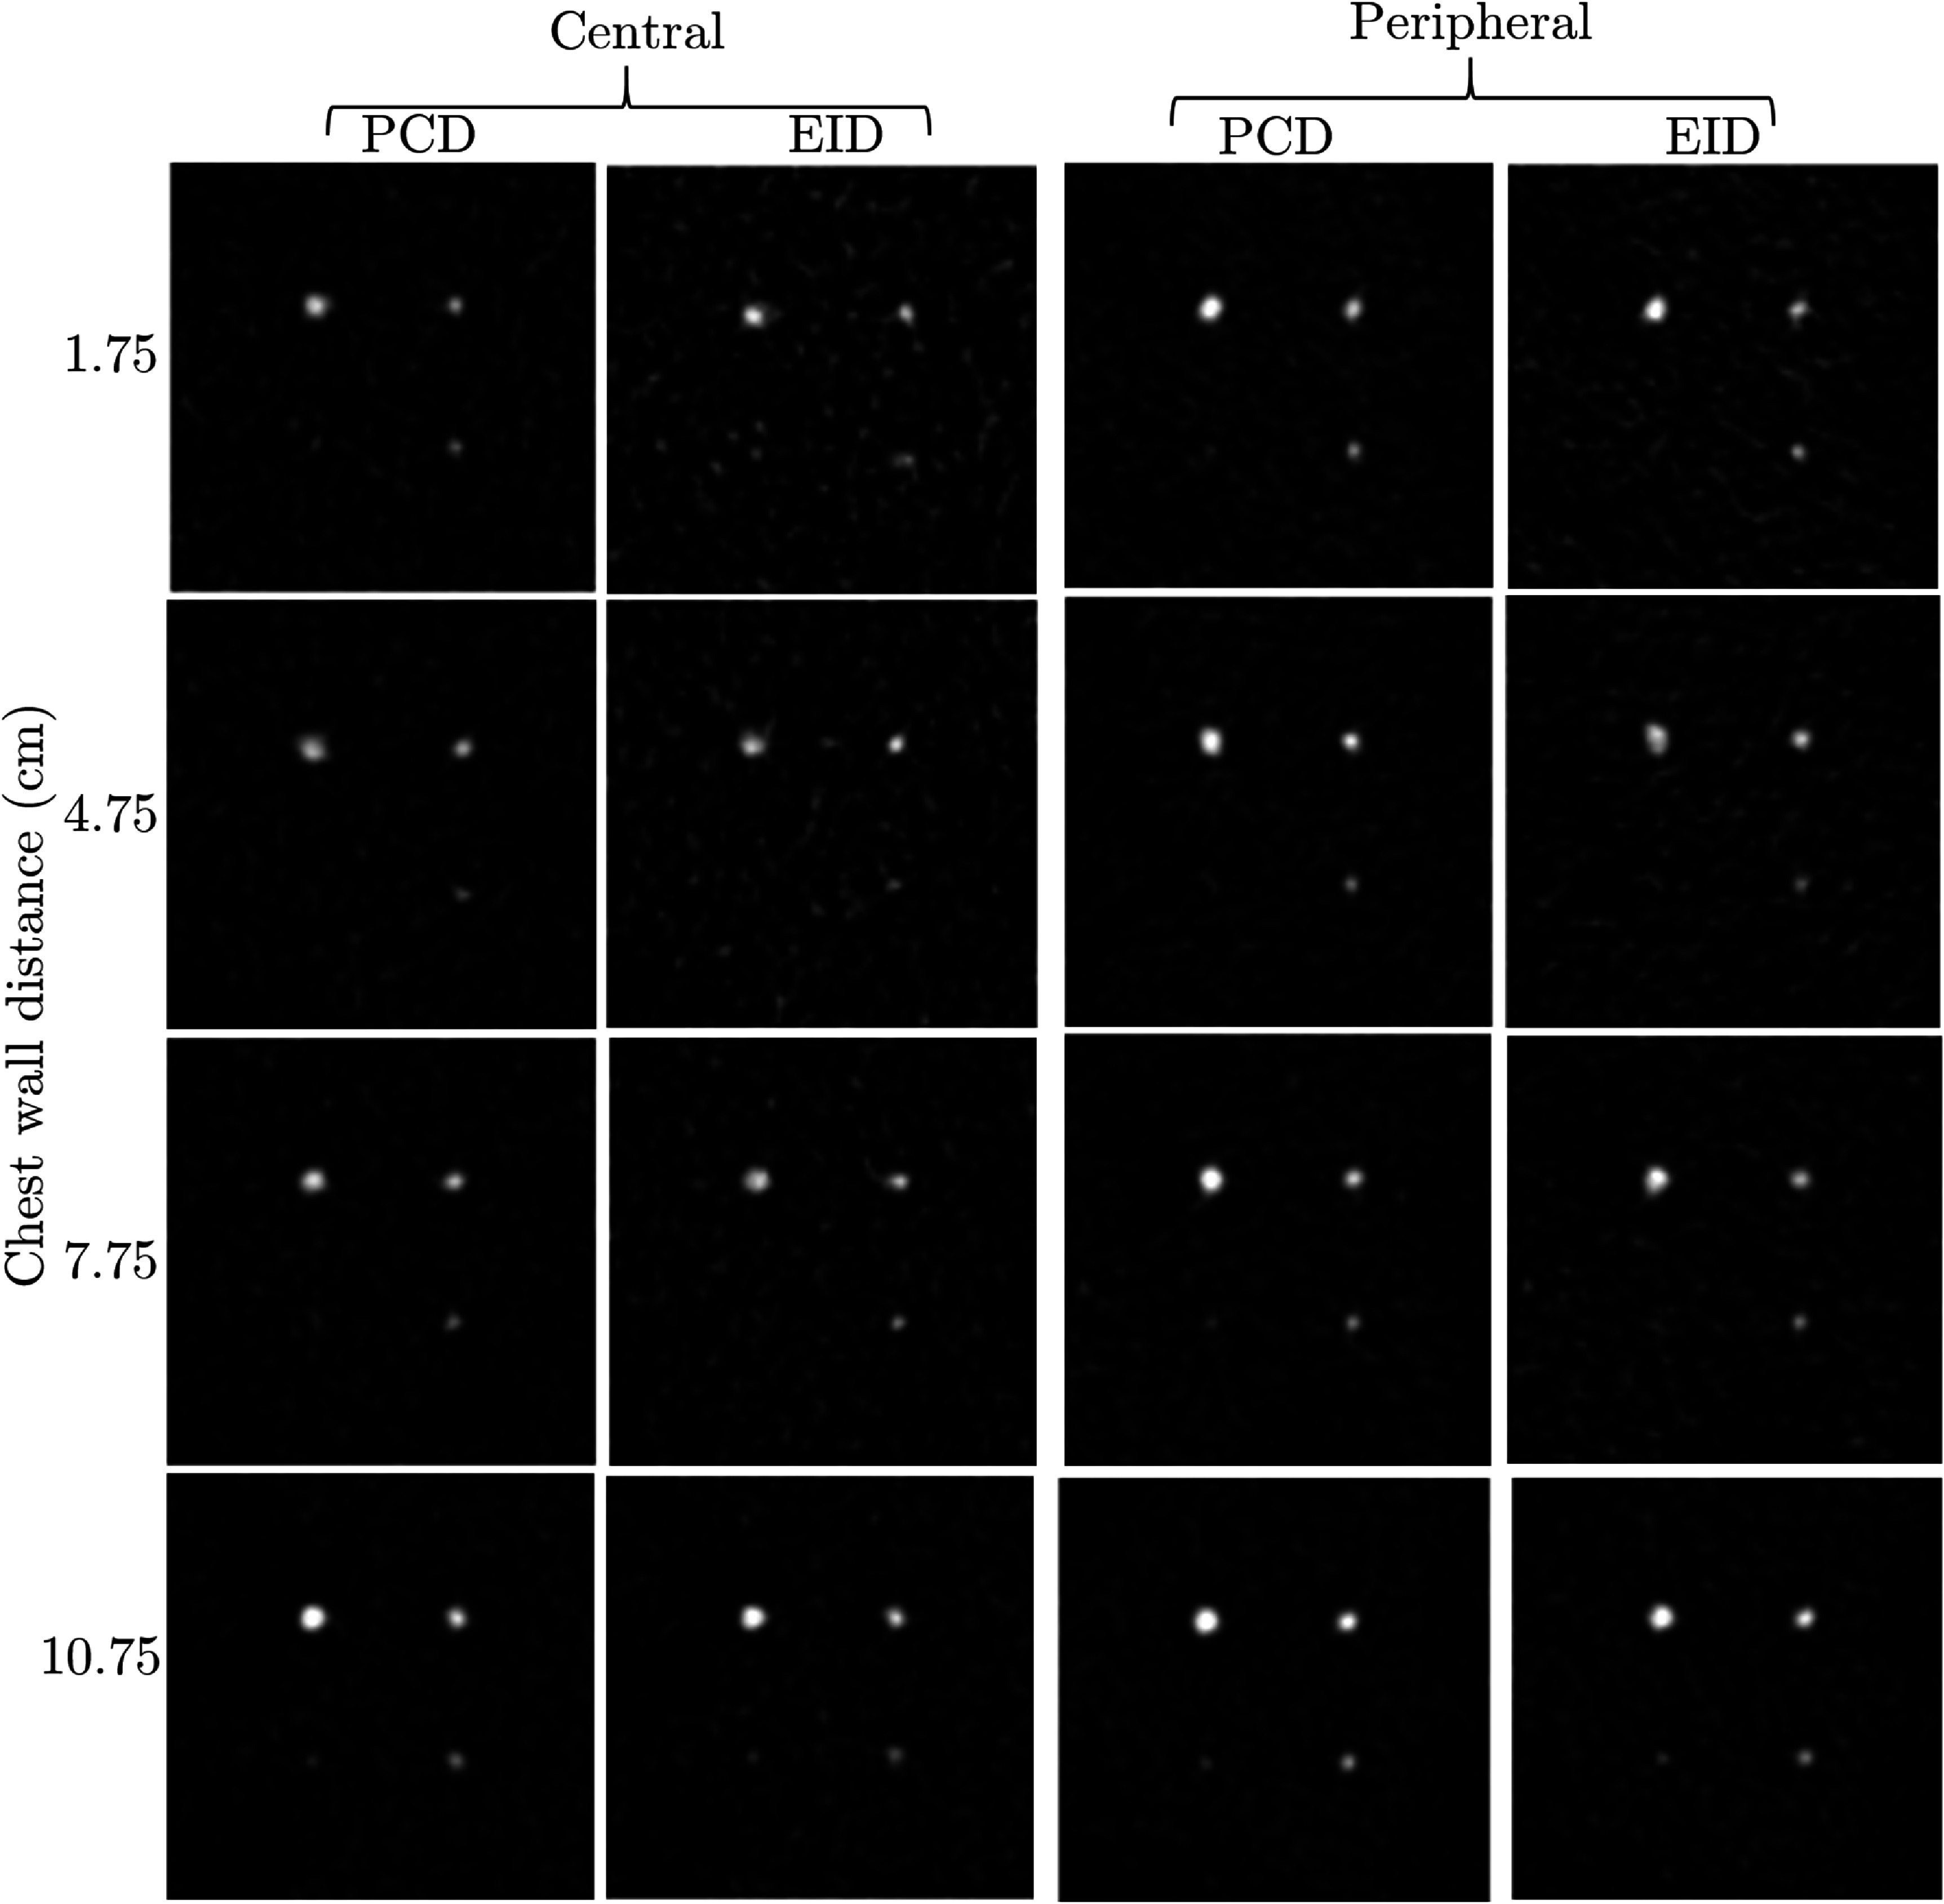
Reconstructed bCBCT images of eight *µ*Calcs clusters at four different distances from the chest wall, and at the central region and the peripheral region of the breast. Display window [0.2, 1.0] cm^−1^.
